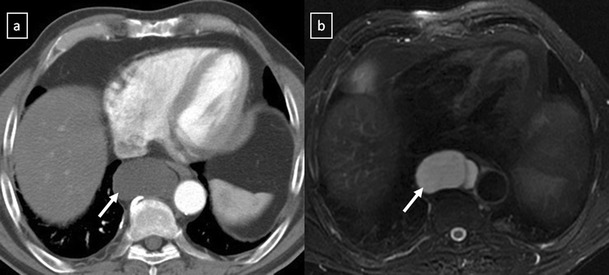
Duplication cyst in a 42-year-old asymptomatic man. a Contrast-enhanced CT scan shows a round well marginated mass (*arrow*) adjacent to the oesophagus with homogeneous water-attenuation. b The lesion (*arrow*) presents a bright signal intensity on T2-weighted fat-suppressed MR image
